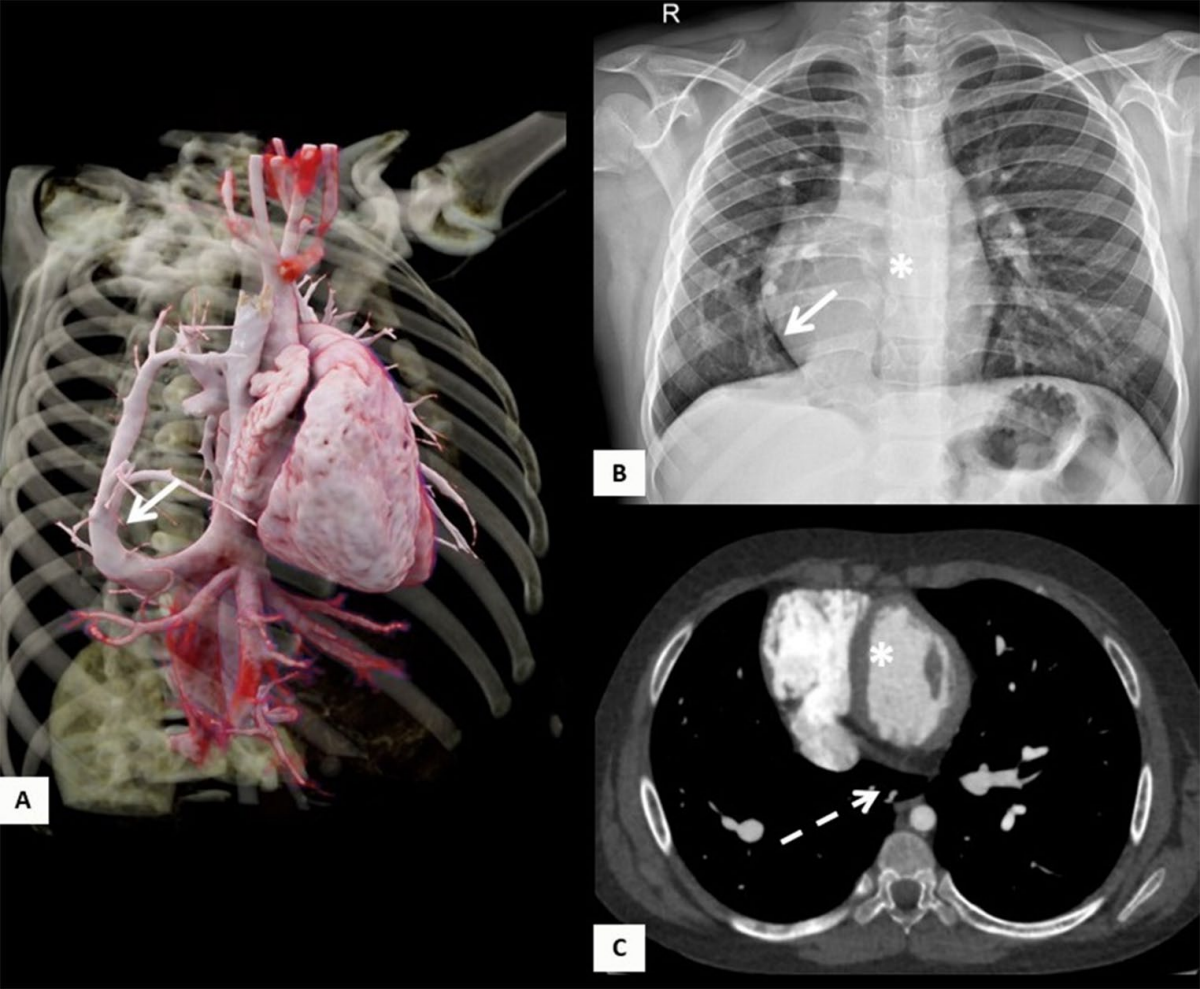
8-year-old male patient with Scimitar syndrome. (A) 3D rendered chest image and (B) chest X-ray, show anomalous right venous drainage into the suprahepatic inferior vena cava (white arrow). (C) Chest CT angiography shows mediastinal midline shift with dextroposition of the heart (asterisk), and horseshoe lung (dashed white arrow)
